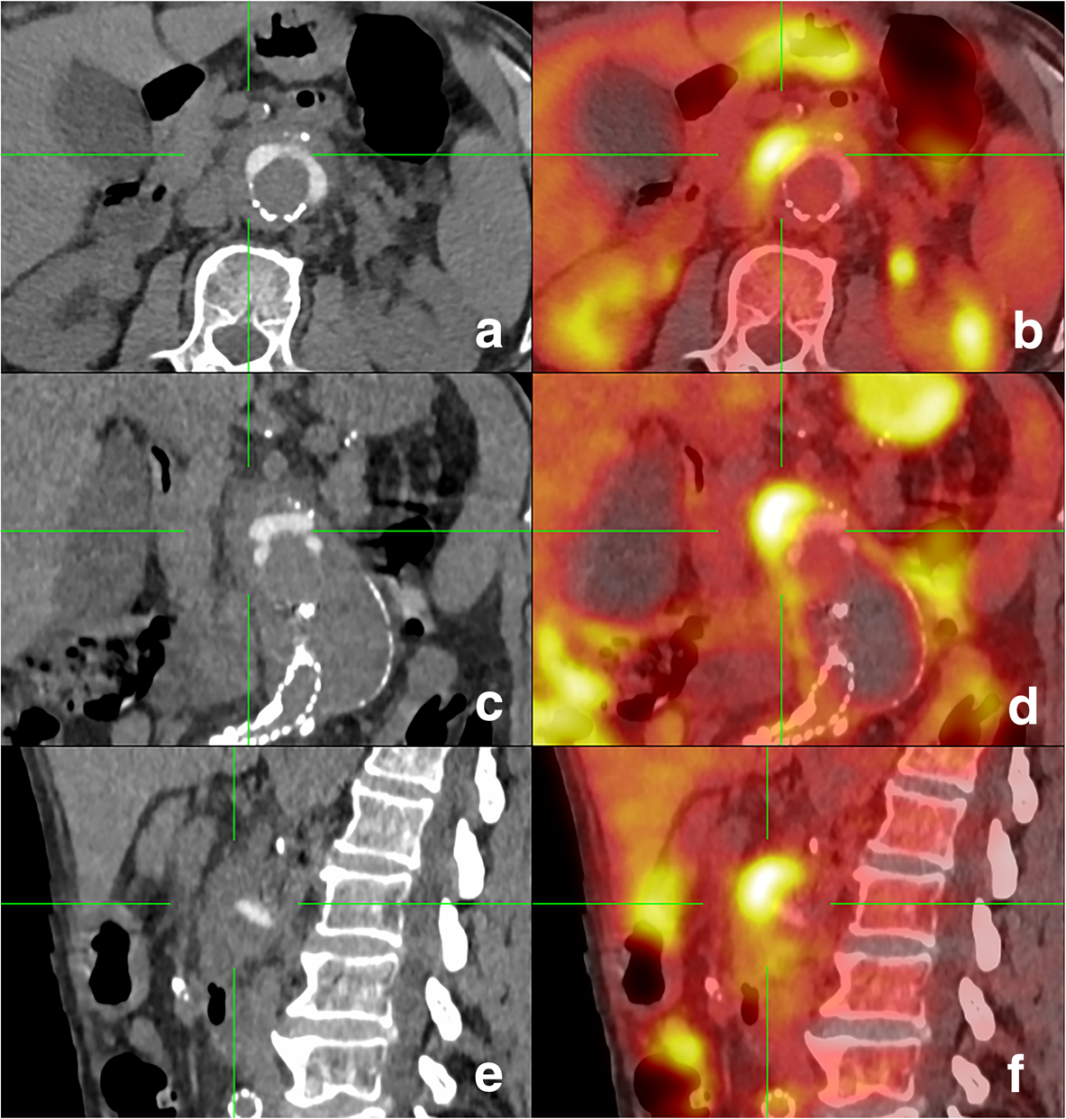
^18^F-FDG PET/CT, axial (a-b), coronal (c-d) and sagittal (e-f) planes. CT shows infiltration of the fat tissues around the aortic endoprosthesis (a, c, e); corresponding PET (b, d, f) reveals an intense ^18^F-FDG hypercaptating mass in contact with the superior antero-medial of the endoprosthesis
